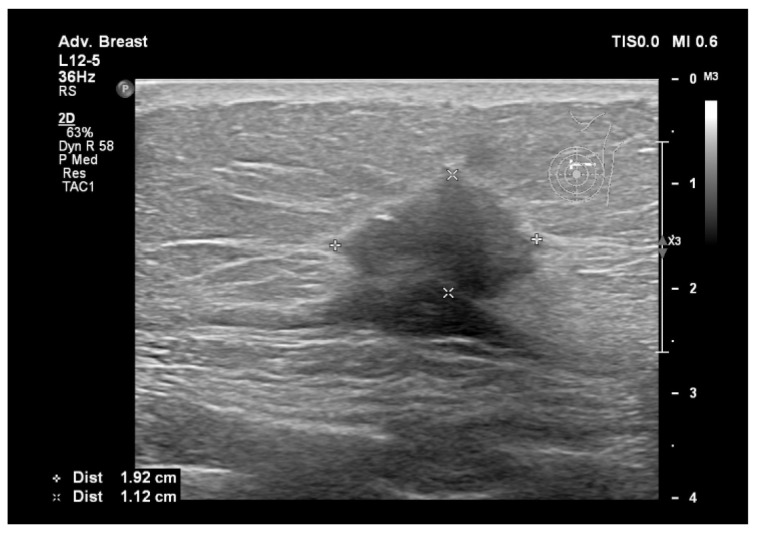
Breast lesion detected using ultrasound, shows a hypoechogenic solid nodule, with spiculations and ill-defined margins.
